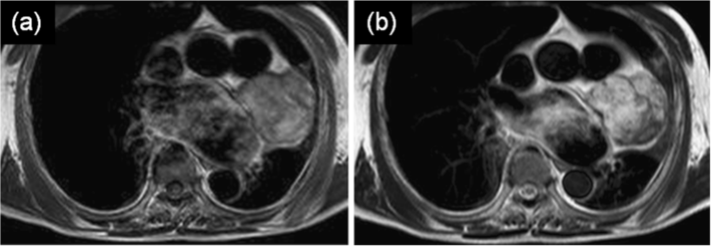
Magnetic resonance imaging. (a) A T1-weighted image (T1WI) demonstrated a well-defined mass of slightly high intensity. (b) A T2WI demonstrated a mixture of high- and low-intensity areas. A low-intensity septum and a peripheral rim were observed in the mass on both T1WI and T2WI.
